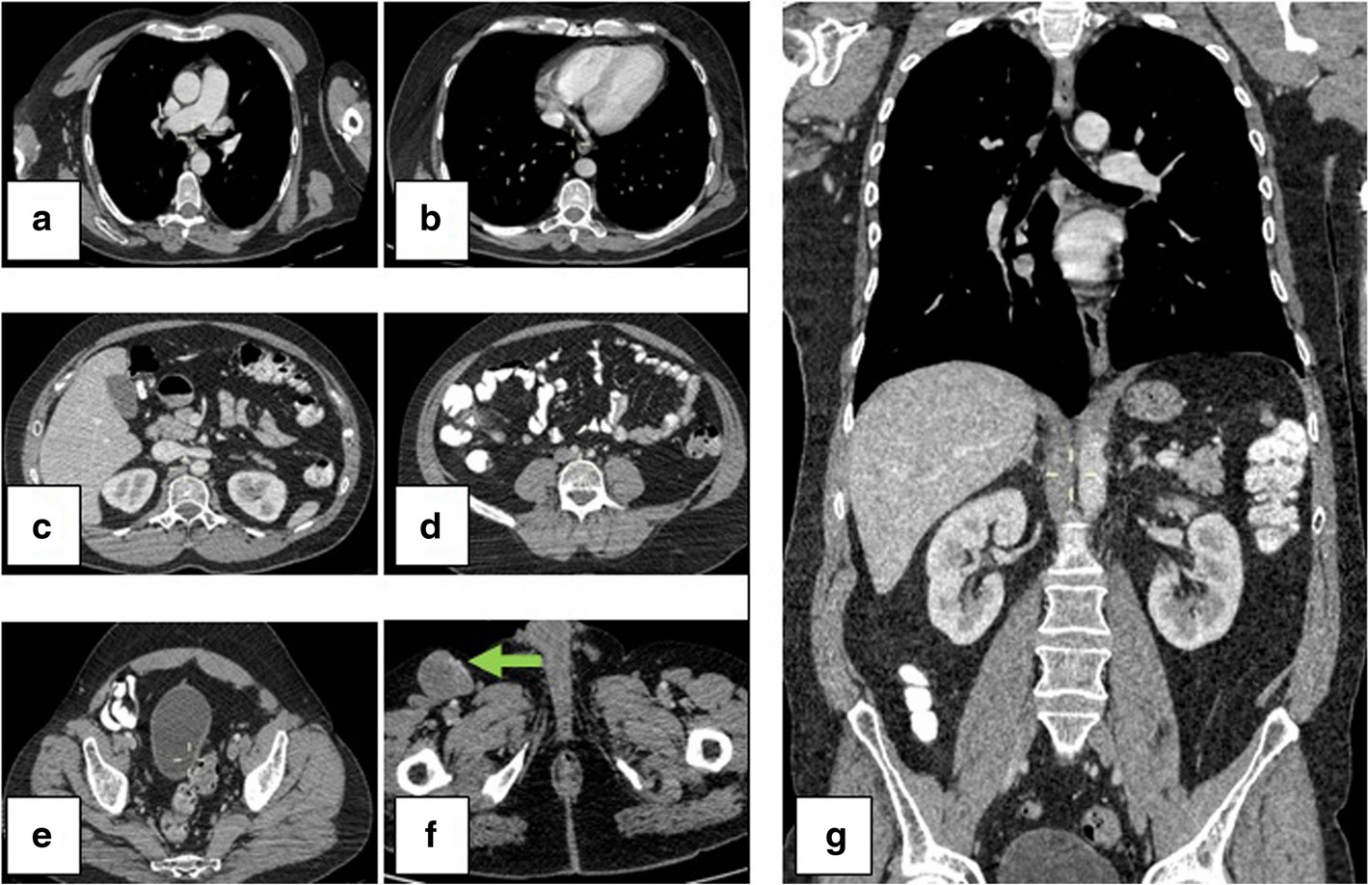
Control CT scan after 17 months of surgery. a–e Axial thoracic and abdominal section without new lesions. f Metastatic right inguinal lymph node, 32 × 22 cm. g Coronal thoracic and abdominal section without lesion
